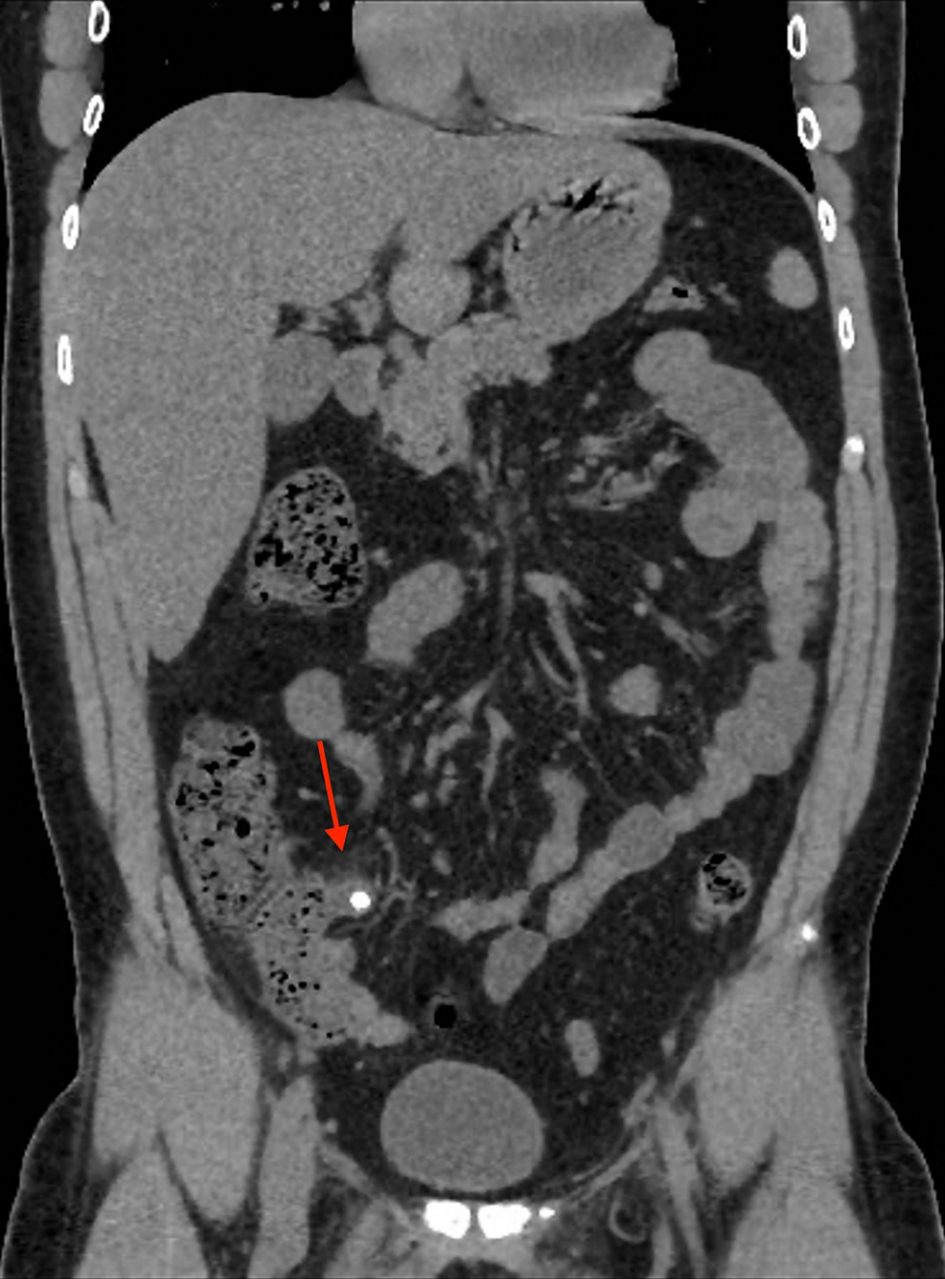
Coronal CT image of the abdomen and pelvis without contrast There is around 1 cm appendicolith seen at the appendices orifice with consequent dilatation of the residual appendix. The diameter of the residual appendix measures about 1.1 X 2 cm. The residual appendix is surrounded by significant fat stranding and multiple reactive regional lymph nodes. There is minimal pelvic free fluid. However, no free air is seen.
